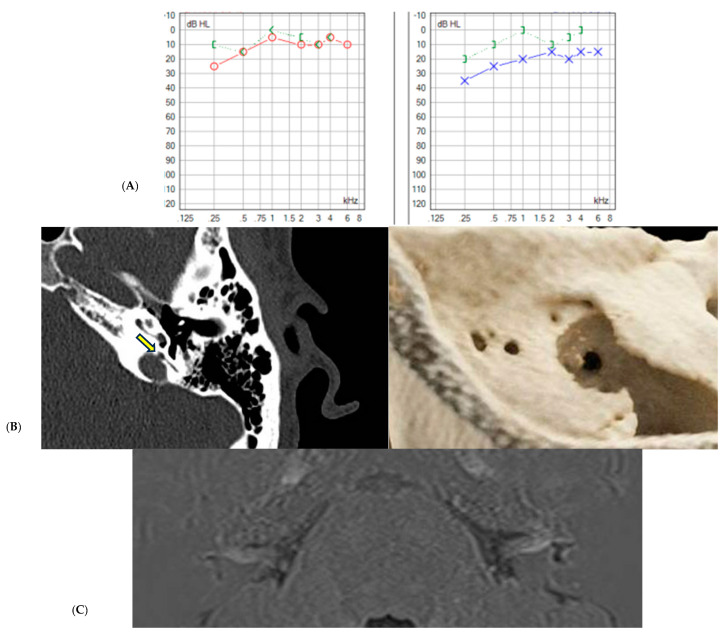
This is the case of an 18-year-old male who presented with loss of consciousness following Tullio and Hennebert phenomena, preceded by otic symptoms and fluctuations in the left ear, as shown in (A), with both bone conduction showed in green and red and blue representing air conduction. A temporal bone CT scan revealed third window syndrome at the level of the ampulla of the posterior semicircular canal involving the jugular bulb, which was later confirmed in a 3D reconstruction, as shown in (B) with yellow arrow. Due to recurrent episodes of vertigo, along with up to three subsequent episodes of fluctuations, an MRI with hydrops sequences (C) was eventually requested, showing dilation of the saccule, confluent with the utricle, indicative of moderate left vestibular hydrops.
